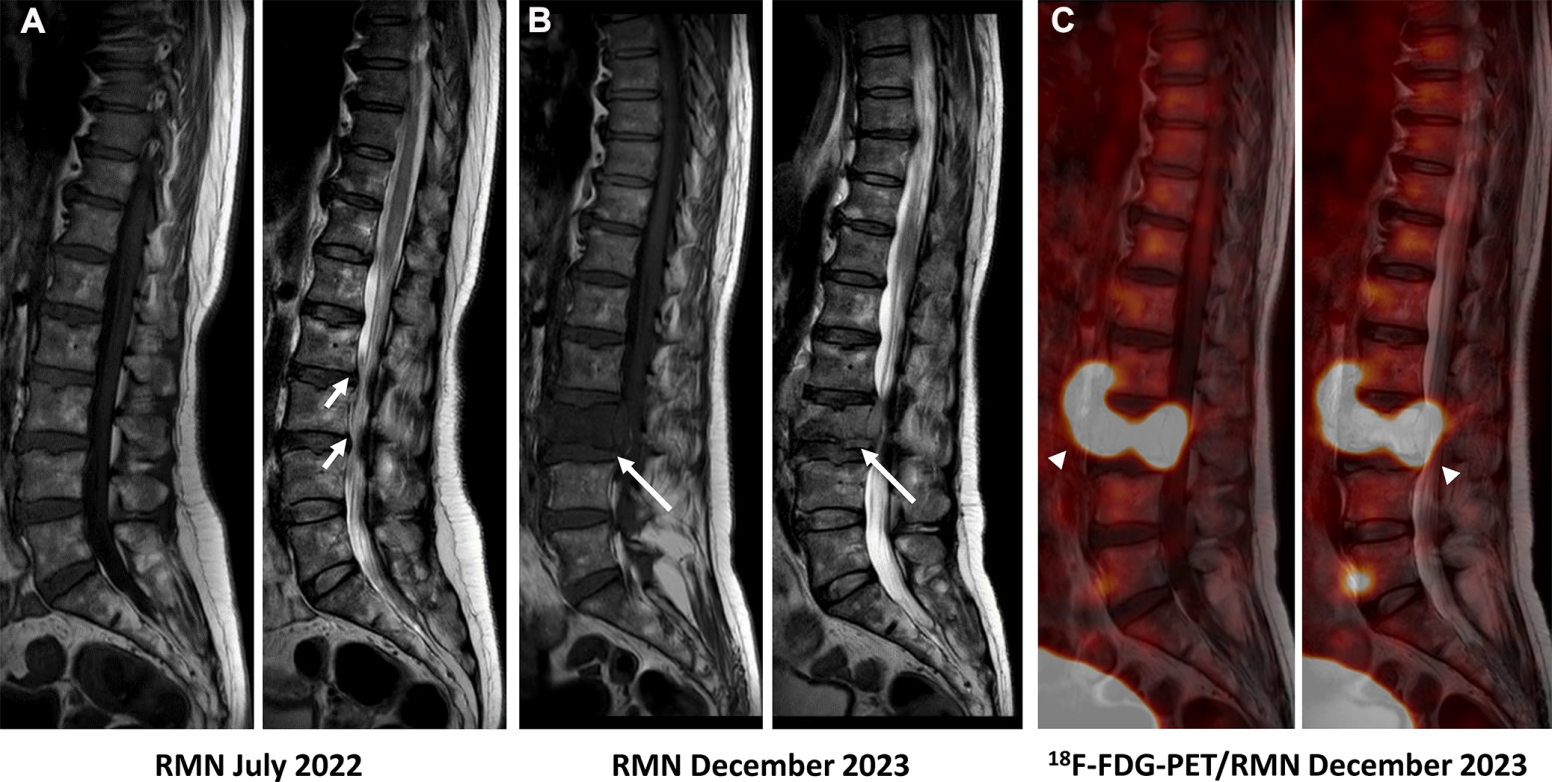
MRI (T1–T2) undergone in July 2022 showed disc disease in L2–L3 and especially L3–L4 (wihte arrow), the latter discreetly reducing the diameter of the canal, with a relative secondary narrowing of the canal. (A) The MRI carried out in December 2023 showed bone marrow replacement almost the entire L3-vertebral body in both phases (long white arrow), and an inflammatory/infectious spindle extending anteriorly and posteriorly, with suspected epiduritis. (B) A merged image of ^18^F-FDG-PET and MRI showed aggressive widespread invasion at the L3 and doubtful at the L3–L4 level intervertebral disc, with an onfectious appearance and metabolic extension anteriorly and posteriorly (head arrow), associated with epiduritis (C).
